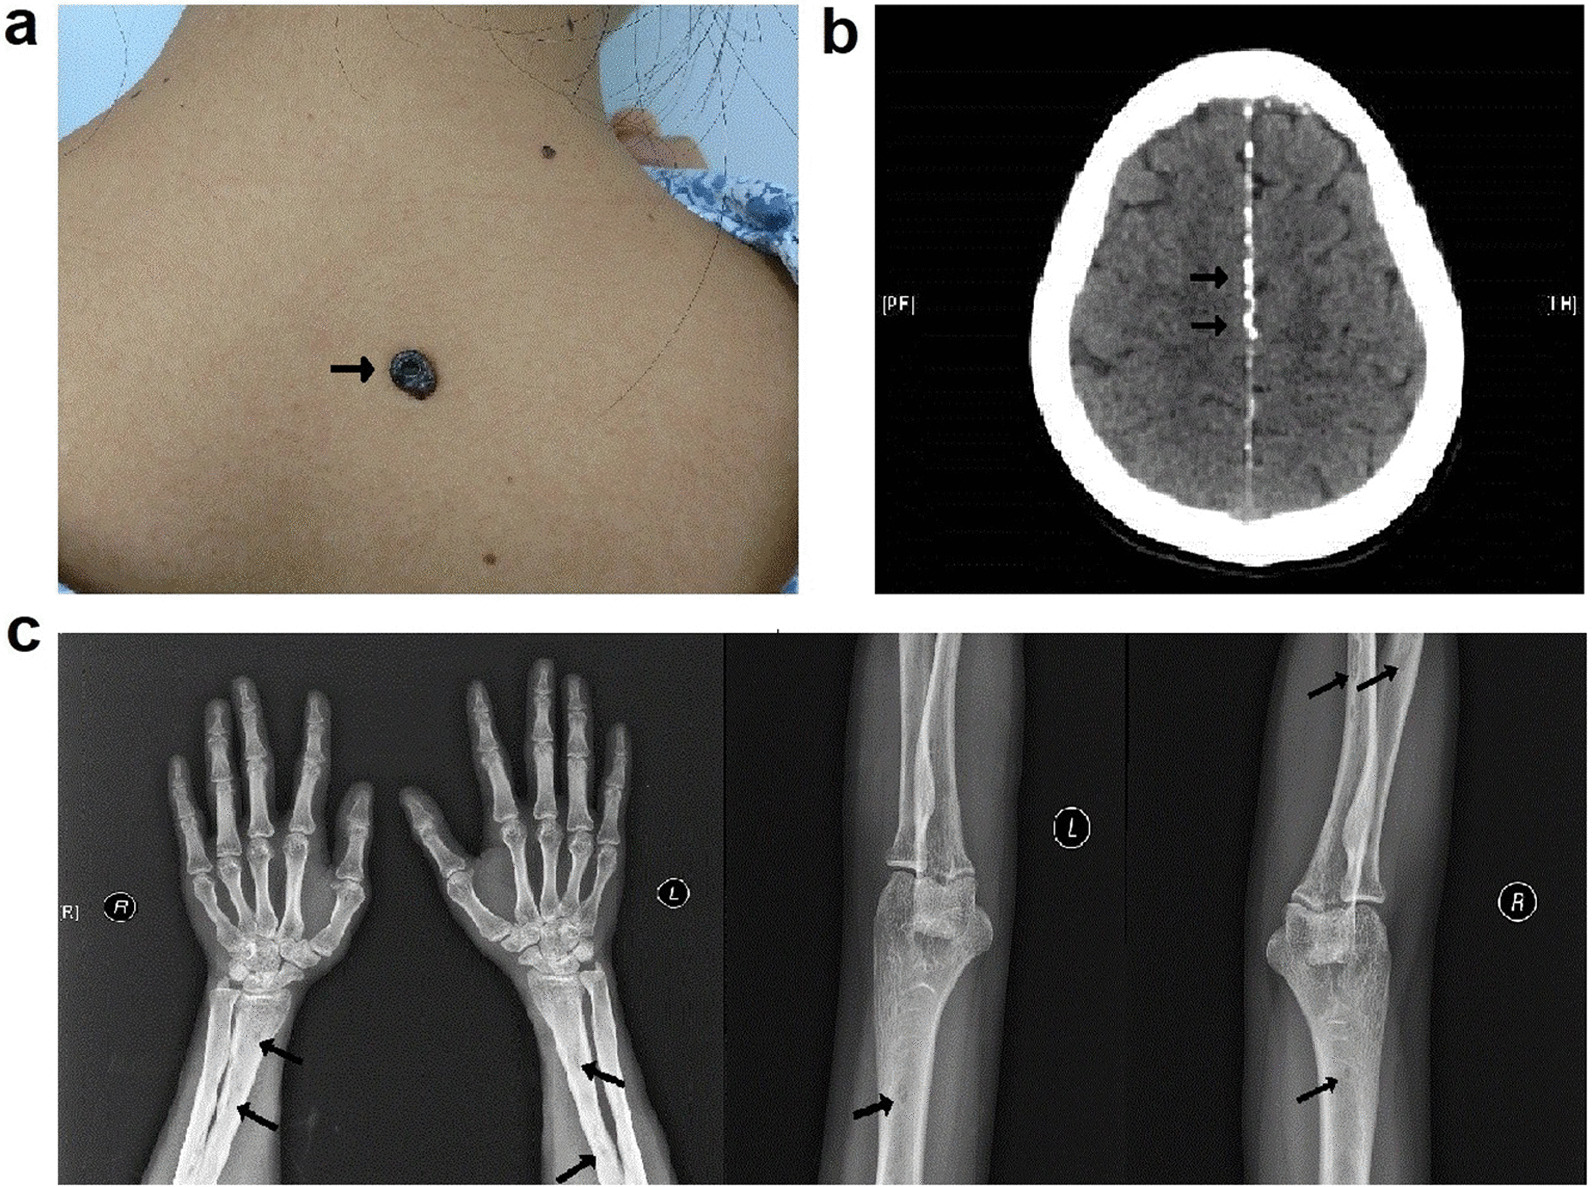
BCC lesions on the back (a), falx cerebri calcification on cranial CT b and low-density shadow on upper-limb X-ray c of the proband, which is indicated by arrows
